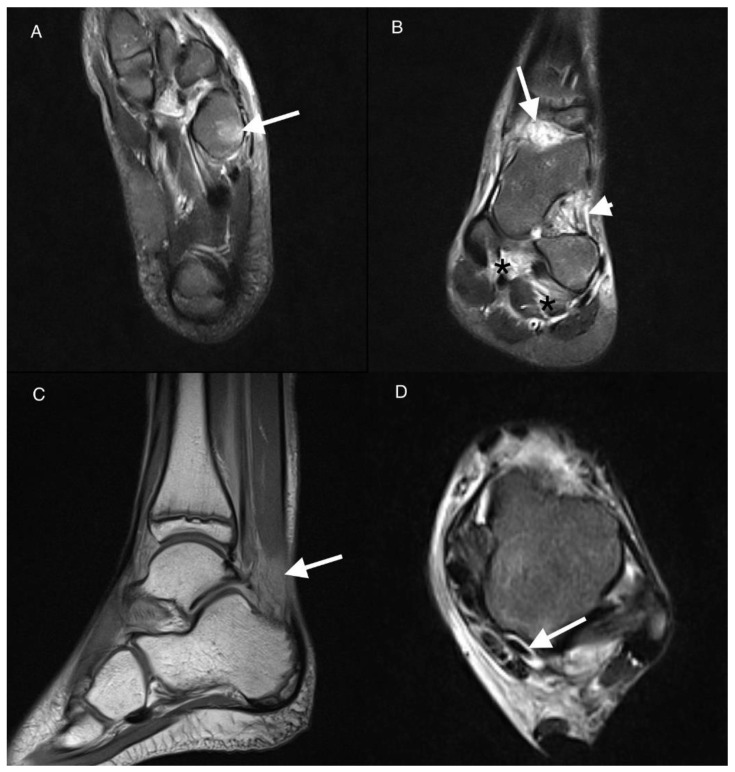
Magnetic resonance imaging (MRI) of the left ankle of an 11-year-old boy with juvenile idiopathic arthritis (JIA). T2-weighted turbo invertion recovery magnitude (TIRM) images in axial (A,D), coronal (B), and sagittal PD-weighted planes (C). Bone marrow edema (BME) stage 2 in the cuboid bone (arrow in (A)). Effusion/synovial thickening stage 2 in the tibio-talar joint (arrow in (B)). Fat tissue in sinus tarsi involvement stage 1 (arrowhead in (B)). Enthesitis of the interosseous ligaments stage 1 (black asterisks in (B)). Kager’s fat pad inflammation arrow in (C). Tenosynovitis of the flexor hallucis longus muscle tendon stage 1 arrow in (D).
